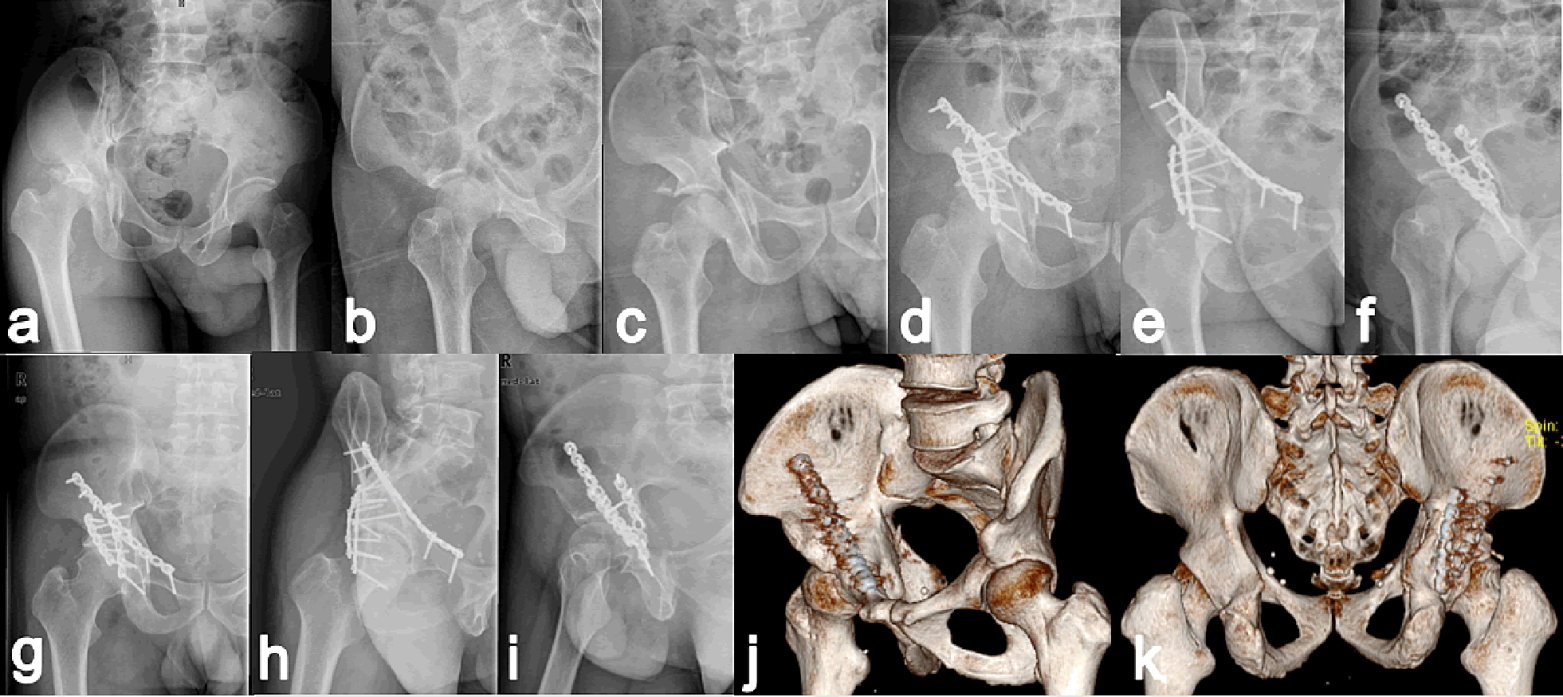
A 43-year-old male treated with KL + IL approaches 5 days after crush injury to hip. a–c Preoperative radiographs revealed T-shaped acetabular fracture involving the posterior wall; d–f Immediate postoperative radiographs showing imperfect reduction; g–k At 9 years of follow up, the patient presented good outcome with slight discomfort of right hip due to mild osteoarthritis, which verified by postoperative images
